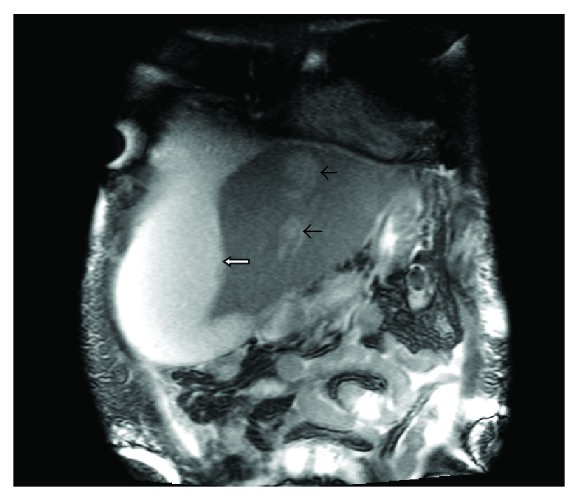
MRI of the abdomen with a large subcapsular abscess and coalescence of hepatic lesions into larger abscesses.
